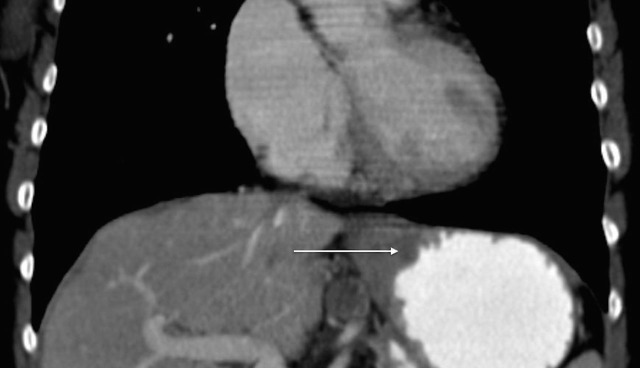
Contrast-enhanced CT image of the abdomen from Patient 2 prior to treatment. This image reveals asymmetric wall thickening of gastric cardia, measuring approximately 2.7 x 4 cm, with stranding into adjacent fat.
